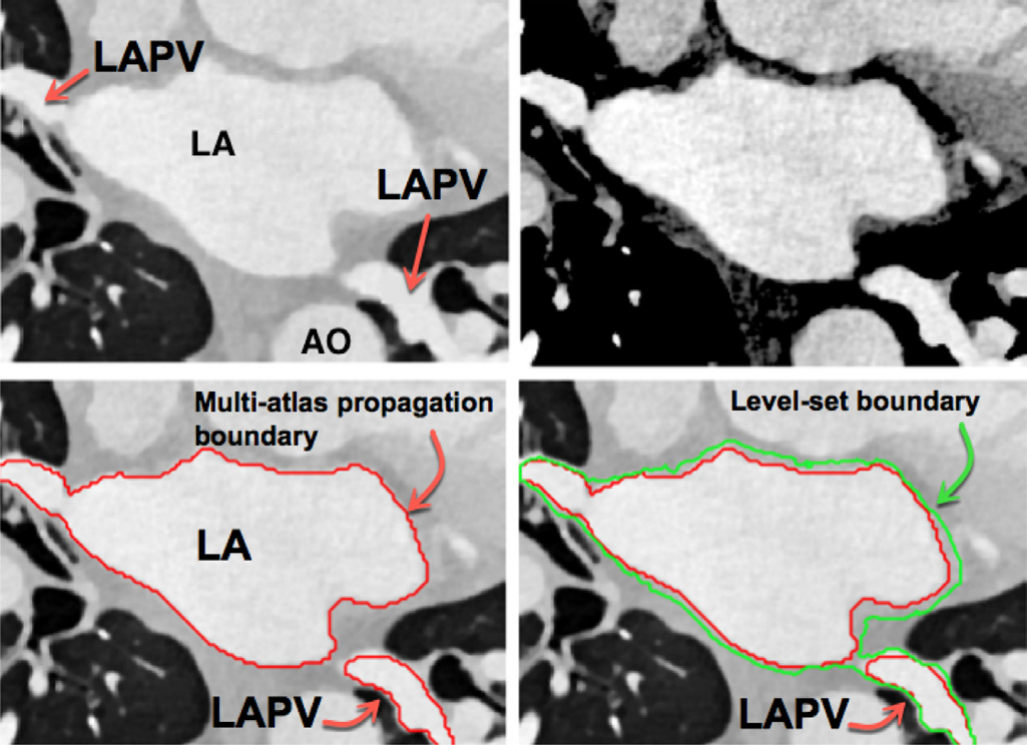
Intermediate steps in the LUMC method. Top row left to right: Original CTA, wall enhancement. Bottom row left to right: inner boundary (in red) obtained with multi-atlas propagation, outer boundary (in green) with the level-set operation. Abbreviations: LA - left atrium, AO - Aorta, LAPV - Pulmonary vein of the left atrium. (For interpretation of the references to colour in this figure legend, the reader is referred to the web version of this article.)
